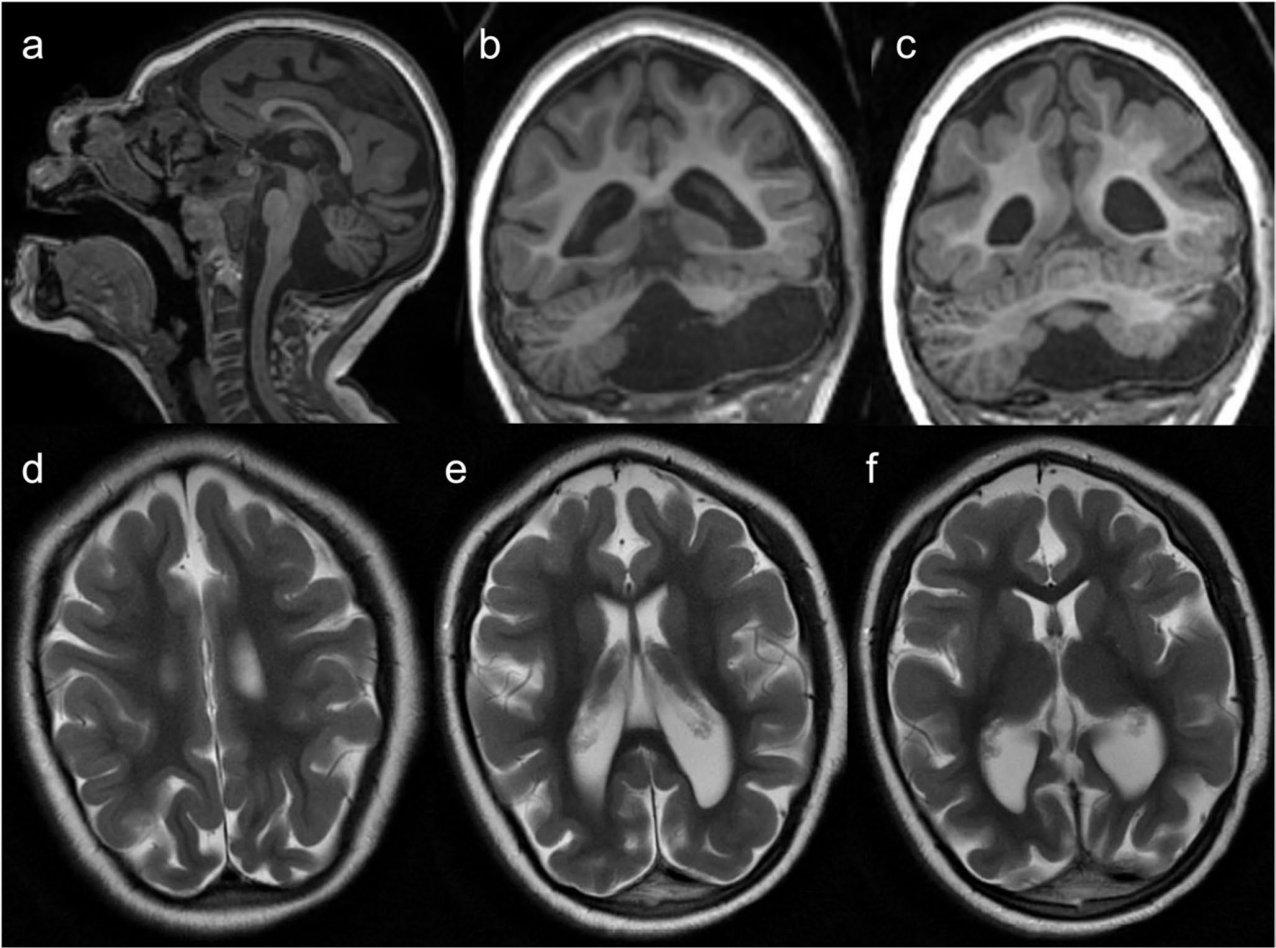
Immunodeficiency, centromeric region instability and facial anomalies syndrome (ICF) type 2. A 5-year-old male patient with a history of epilepsy, cerebral palsy, microcephaly, and facial anomalies (micrognathia, macroglossia, low-set ears, hypertelorism, and epicanthal folds) presenting with EBV-associated polymorphic T-cell lymphoproliferative disease. Next-generation sequencing panel of EBV-susceptibility related genes identified a homozygous ZBTB24 mutation. Brain MRI (a – sagittal T1, b and c – coronal T1, and d to f – axial T2) demonstrates microcephaly with a simplified gyral pattern, upward displacement of the hypoplastic left cerebellar hemisphere, and asymmetric dilation of the fourth ventricle, connected to an ipsilateral retrocerebellar cyst. These findings resemble the ‘tilted telephone receiver’ sign, characteristic of PHACES syndrome
